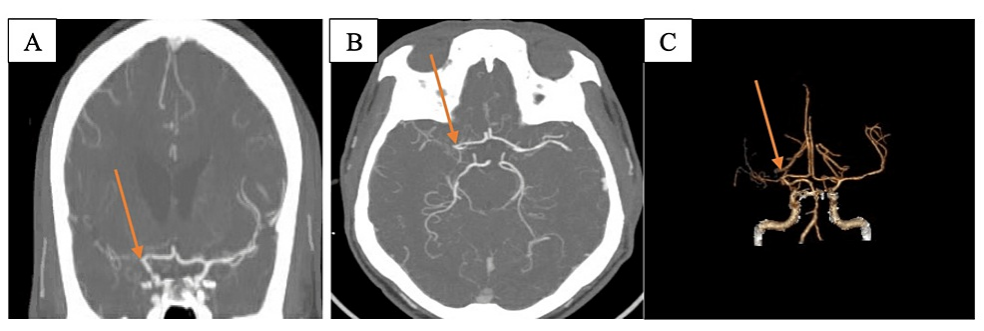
(A-C) CT angiography revealed marked attenuation of the terminus of the right supraclinoid internal carotid artery and near occlusion of the right middle cerebral artery (arrows).
